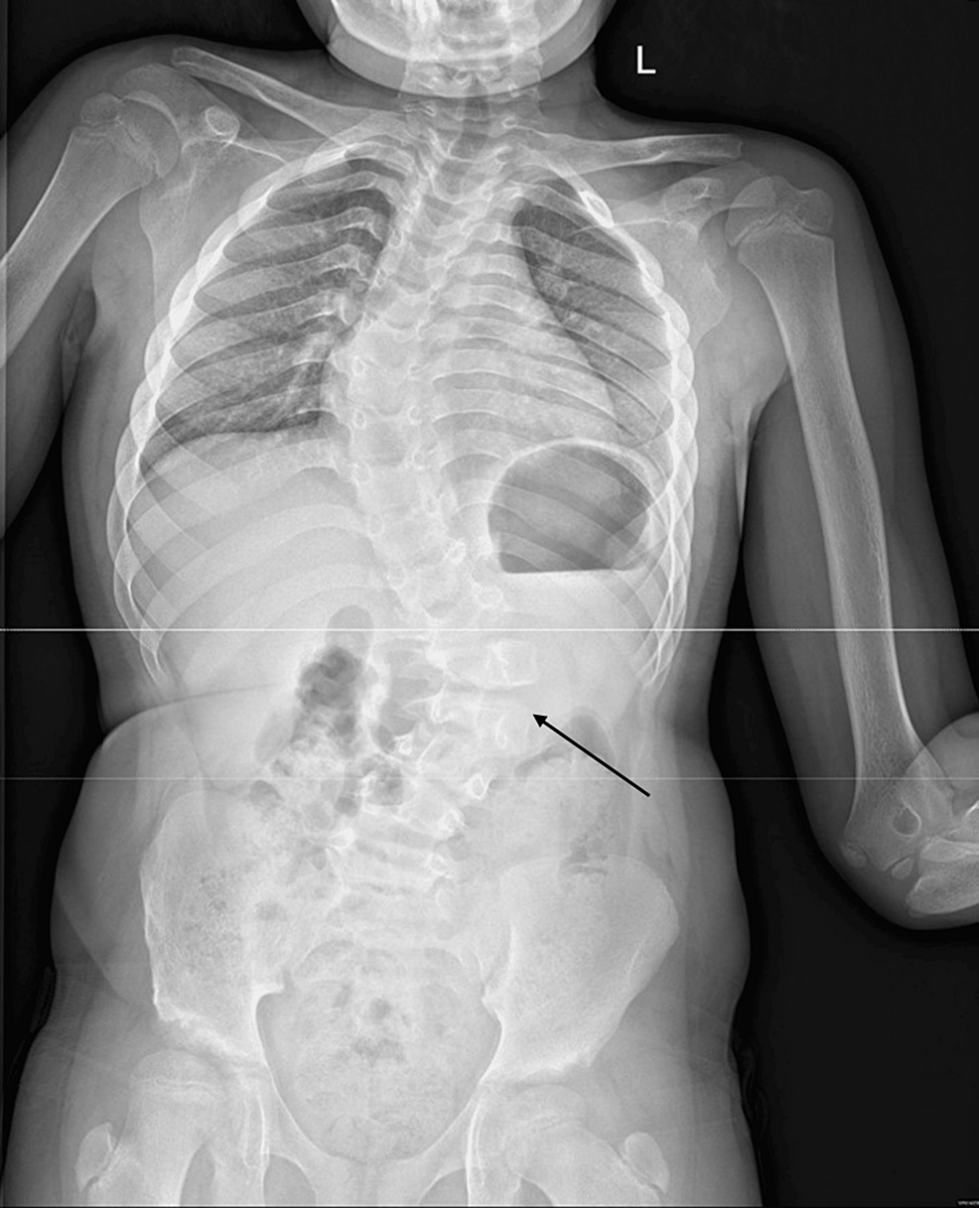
X-ray of the whole spine shows re-demonstration of S-shaped scoliosis. The dorsal dextroscoliosis Cobb's angle measures approximately 47 degrees; the lumbar levoscoliosis Cobb's angle measures approximately 35.7 degrees.
